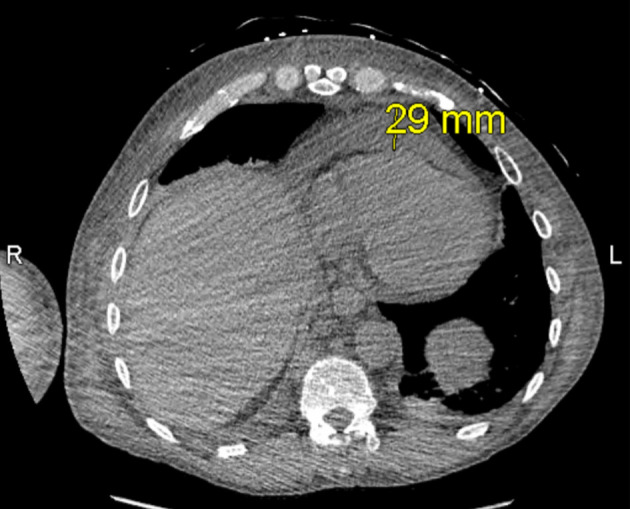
Computed tomography (CT) ion scan of the chest obtained on hospital day 4, depicting pericardial effusion.
